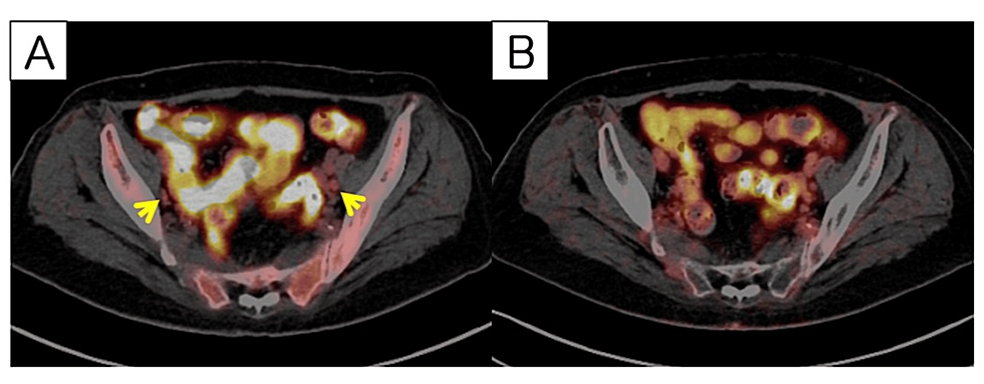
FDG-PET/CT images at initial diagnosis and after systemic TP chemotherapy FDG-PET/CT images at initial diagnosis are shown in 3A and after systemic TP chemotherapy are shown in 3B. The yellow-colored arrowheads indicate metastatic pelvic lymph nodes with moderate accumulations of FDGs, and these abnormal accumulations disappeared after chemotherapy.
